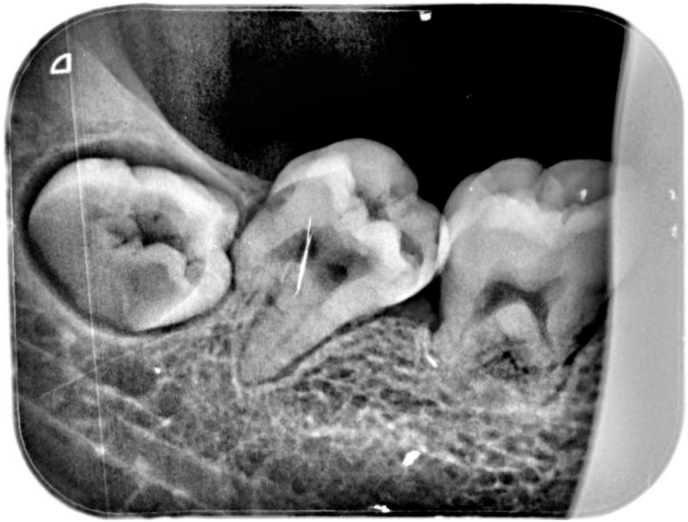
Periapical X-ray showing shortened roots with mesial enlargement of periodontal ligament of 47.
